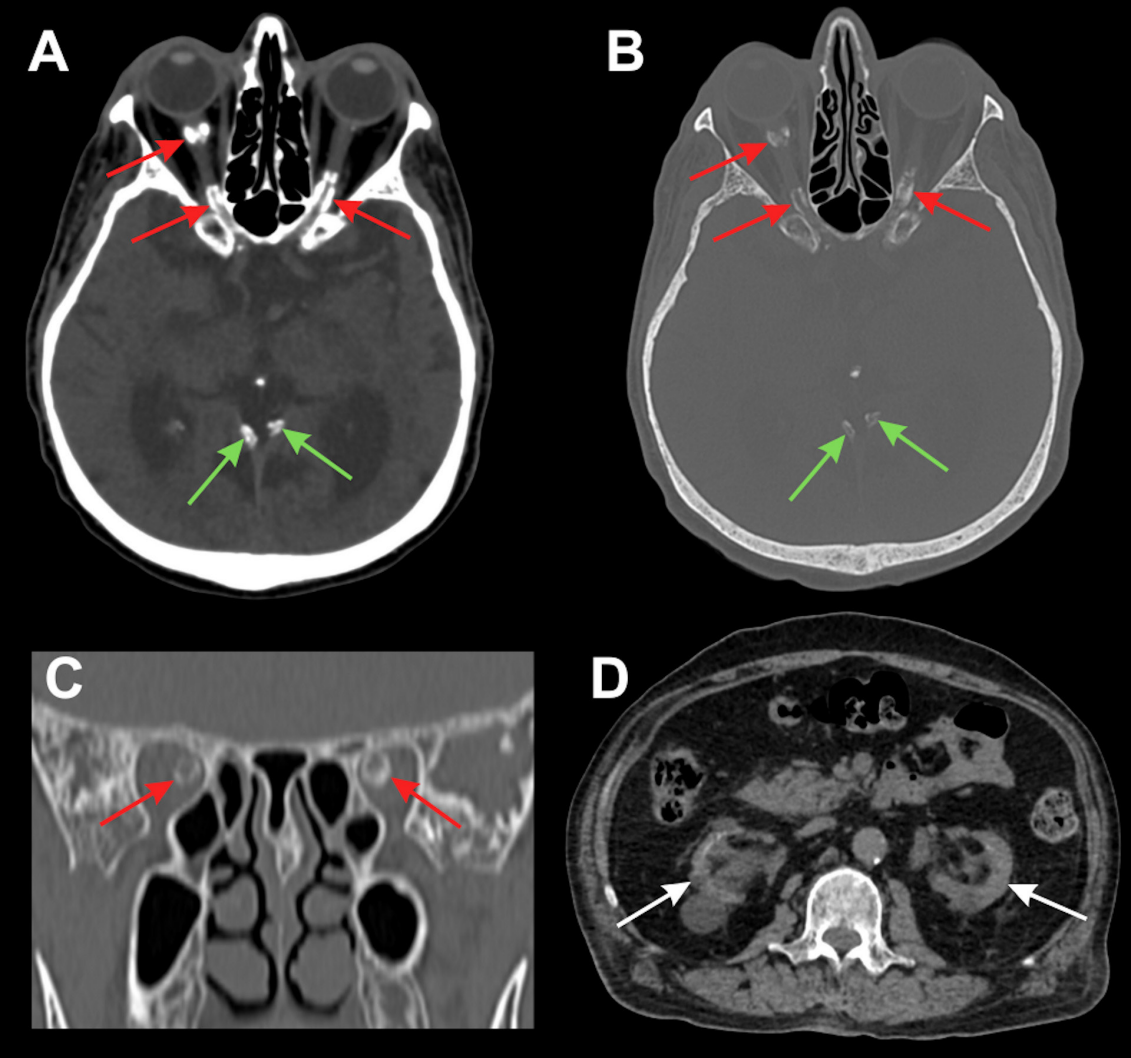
Optic nerve sheath calcifications due to hyperparathyroidism secondary to chronic kidney disease. Brain computed tomography (CT) revealed bilateral calcific foci in the optic nerve sheaths (arrows in A, B, and C), in the brain parenchyma window (A), and in the bone window (B and C). Meningeal calcifications were also noted in the tentorium cerebelli (green arrows in A and B). Imaging evaluation of the kidneys on upper abdominal CT showed a marked reduction in renal parenchymal thickness and multiple bilateral cortical cysts (arrows in D), in addition to some aortic calcifications.
